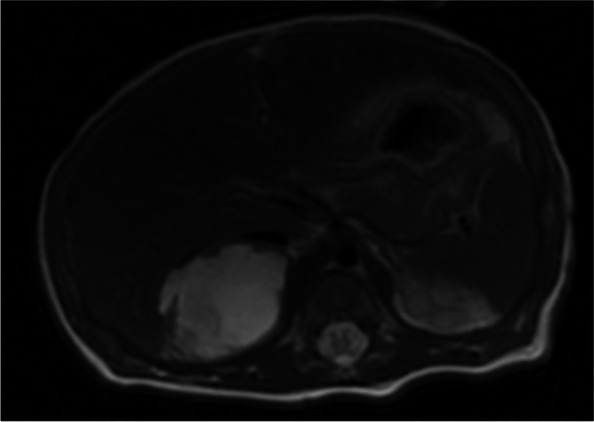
Abdominal MRI showed the adrenal hemorrhage(3.1*3.6 cm)
